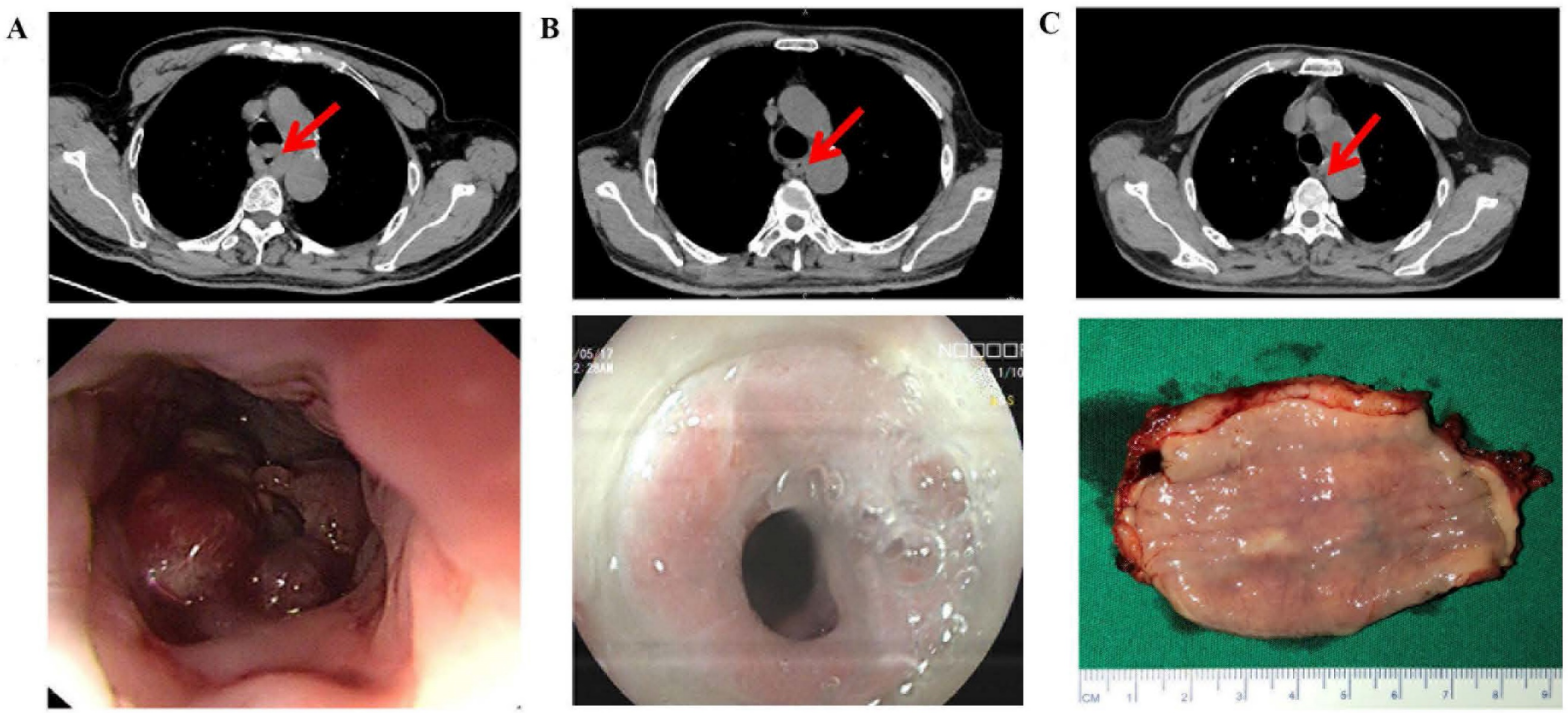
Radiographic response leading to pCR after three cycles of neoadjuvant therapy (top: cross section, bottom: gross view). A: Before treatment. B: tumor diameter regressed by 50% after the second. C: Tumor disappeared after the third cycle.
